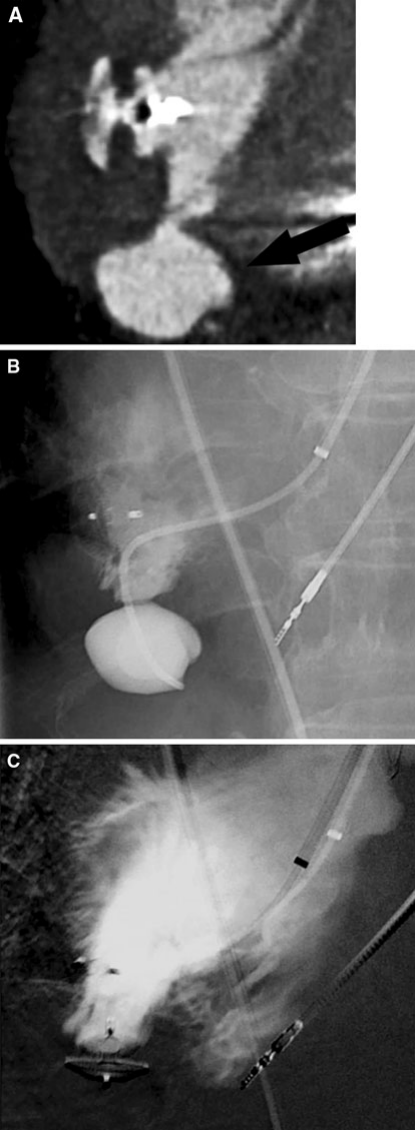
Second pseudoaneurysm at the bottom of the apex. A CT after 1 week demonstrates exclusion of the first pseudoaneurysm and the second aneurysm (*arrow*). B Angiography catheter tip positioned in the second pseudoaneurysm. C The second pseudoaneurysm excluded with the amplatzer
